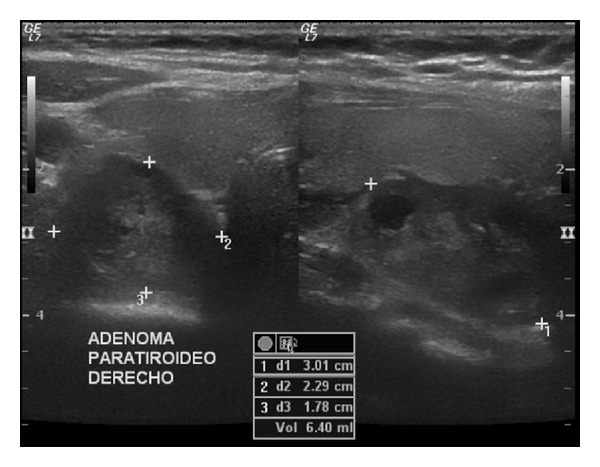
Right inferior parathyroid gland (ultrasonography).
